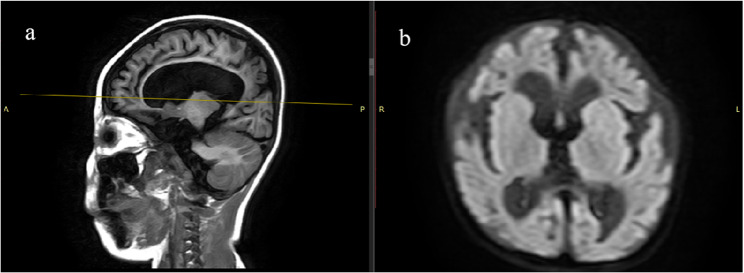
a The MRI reveals cerebral atrophy with reduced white matter volume, ventricular dilation, and thinning of the corpus callosum. b DWI showed resolution of the abnormal signals in the bilateral basal ganglia, thalamus, and corpus callosum
